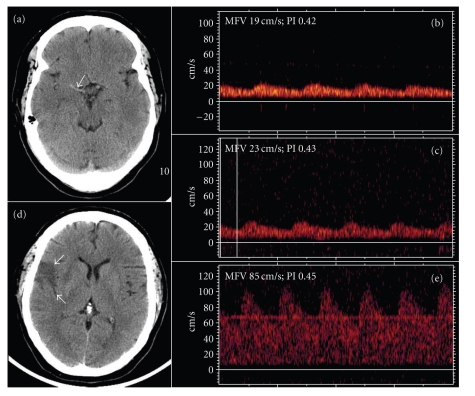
(a) Baseline noncontrast CT: a long thromboembolus within the right MCA trunk (arrow). (b) Baseline TCD: flattened systolic flow acceleration (TIBI 2) at a depth of 48 mm, indicating right proximal MCA occlusion. (c) One-hour TCD: improved flow velocities/waveforms (TIBI 2) at a depth of 58 mm suggestive of slow and partial recanalization. (d) Followup CT reveals a small cortical infarction (arrows). (e) 24-hour TCD: normal flow velocities and waveforms (TIBI 5) at a depth of 46 mm.
